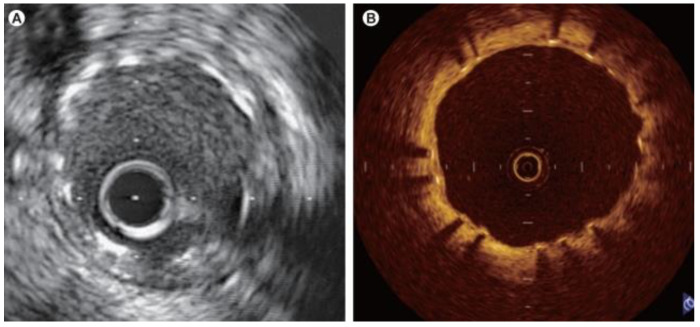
Images obtained at 7 months after implantation of a drug-eluting stent (Cypher; 3.0 mm × 18 mm) implantation: (A) IVUS, intravascular ultrasonic; (B) OCT, optical coherence tomography. Reproduced under a Creative Commons license from [115].
